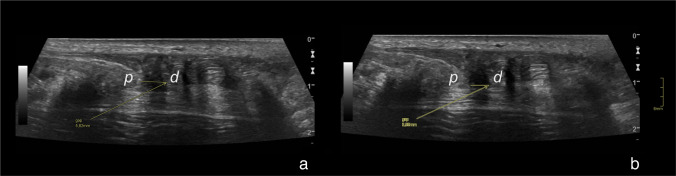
Ultrasound long-axis image demonstrating discordant tendon gap measurement between two raters. Differences in frame selection and/or caliper placement across irregular tendon stump margins resulted in different gap measurements (a—Rater 1: 5.8 mm; b—Rater 2: 4.8 mm). These measurements fall on opposite sides of the 5—mm threshold and would therefore classify the patient into different treatment groups within a protocol-based pathway. *p* = proximal stump; d = distal stump
